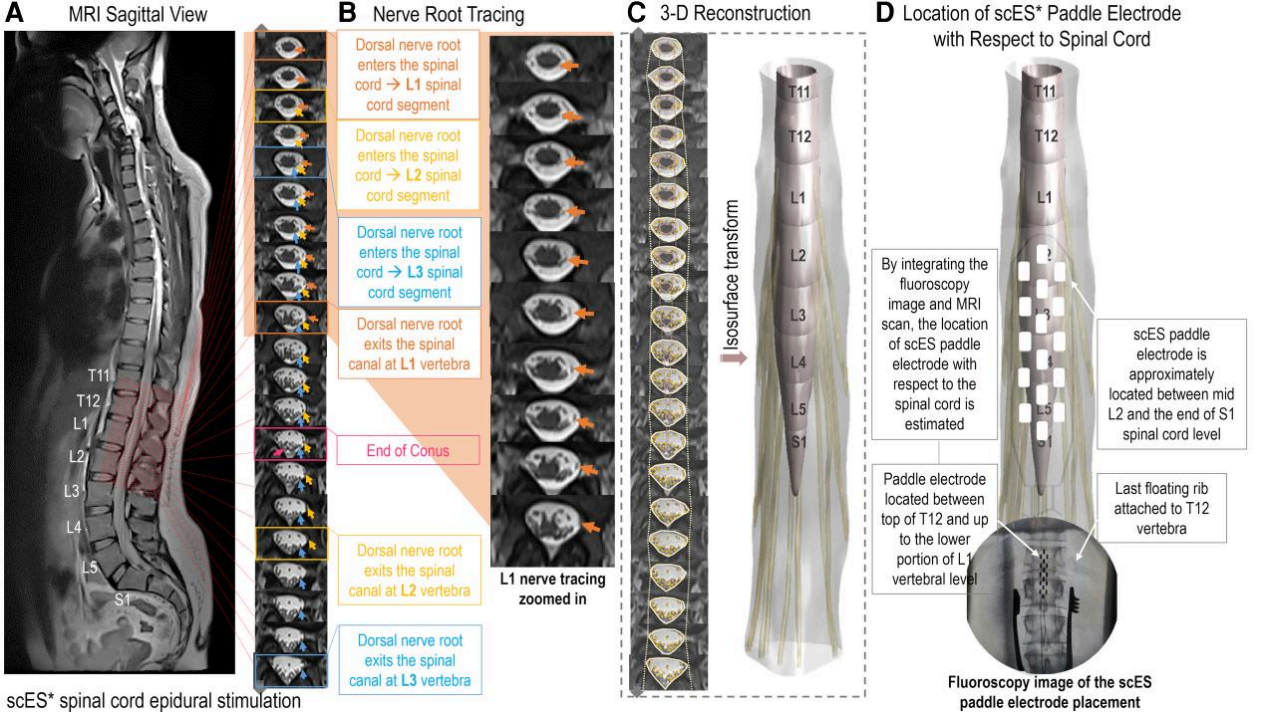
Neuroanatomical mapping of the spinal cord at LSE. (A) MRI sagittal view of the whole spine in an individual with a cervical spinal cord injury. (B) Axial images of the lumbosacral region from high spatial resolution MRI recording, with the arrows pointing at the dorsal nerve roots from the vertebral site of the exit back to the location of entering the spinal cord to show how spinal cord levels are identified using the nerve root tracing technique. (C) Process of tracing and labelling the spinal cord region, cerebrospinal canal and nerve roots in each axial image and using the isosurface function to build a reconstructed model of the lumbosacral spinal cord from the segmented images. (D) Process of integrating intraoperative fluoroscopy images of the paddle placement with the preoperative MRI recordings in order to estimate the location of the paddle array on the spinal cord model.
